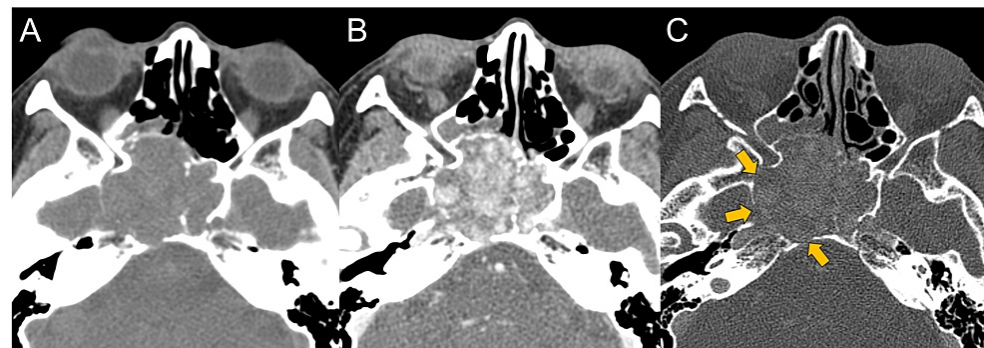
Non-contrast and contrast-enhanced computed tomography at the time of the visit On a computed tomography (CT) scan, the mass shows expansive growth and iso-density on non-contrast CT (A). Contrast-enhanced CT in the arterial dominant phase revealed enhanced vessel-like structures within the lesion (B). There is remodeling rather than destruction of the surrounding bones (C, yellow arrows).
